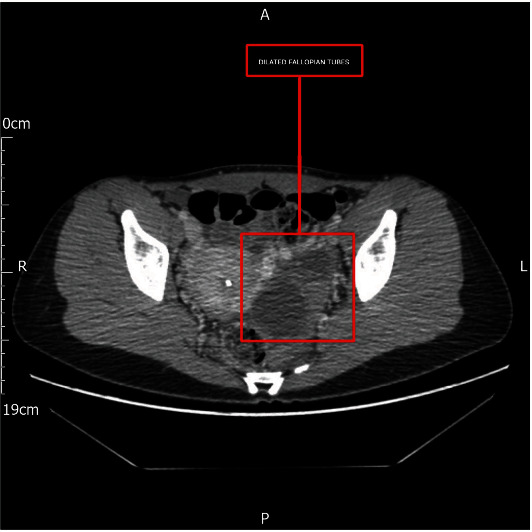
CT scan images. CT scan impression: −7 × 8 cm cystic structure noted in the left adnexa—tubo-ovarian abscess hydrosalpinx.
